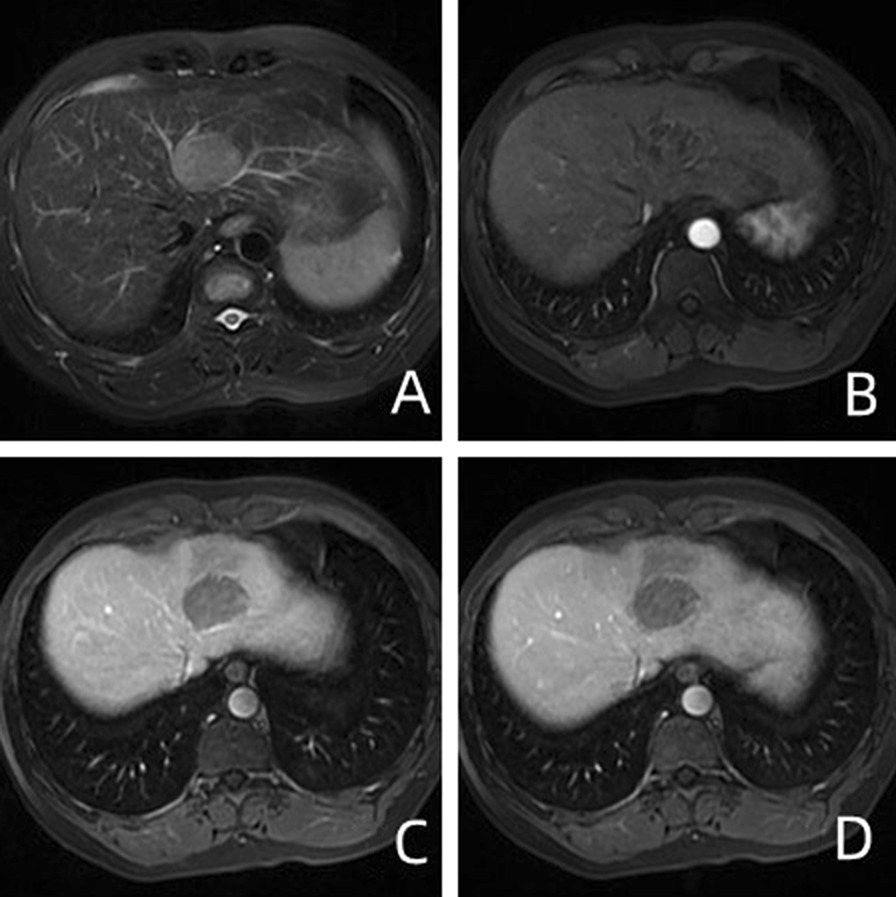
A 50-year-old man presented with low-grade HCC with MVI. A Unevenly high signals on T2WI; B, C Heterogeneous enhancement (black arrow) in the left lobe of liver in the arterial and delayed phases of GD-BOPTA–enhanced MRI; D Non-smooth tumor edge and peritumoral hypo-signal intensity (white arrow) in the hepatobiliary phase
